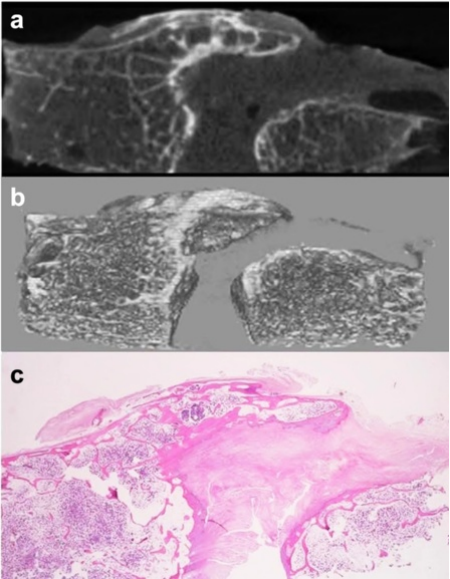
a–c Representative case of the continuous posterior-annular fibrosus type. a CT image: A trabecular bone structure was frequently observed within the high-density area. b Three dimensional reconstruction image: The high-density area was observed as an elongation of the rim of the vertebral body. c Pathological image: These cases typically involved a projection with continuity with both marrow and the cortex of the bone to the enthesis. Calcification was observed in the connective tissue around the projection
